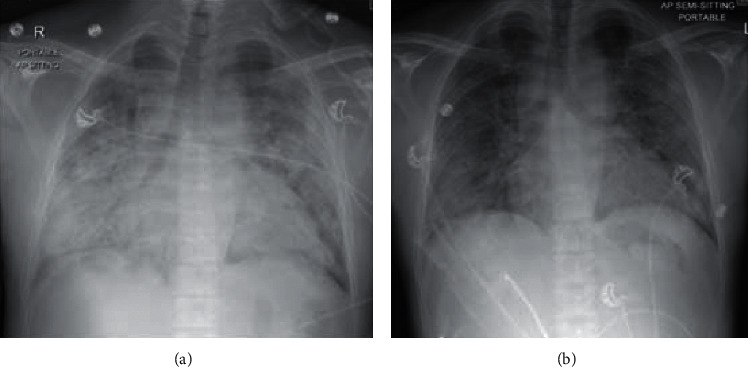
Chest X-ray AP view showing extensive bilateral perihilar and basal opacities on day 2 (a), which has improved significantly by day 4 as shown in (b).
